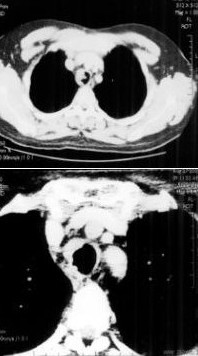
a) Chest CT scan displays a polypoid mass occupying 50 % of the lumen. b) Control CT scan displays resolution of the tumor
